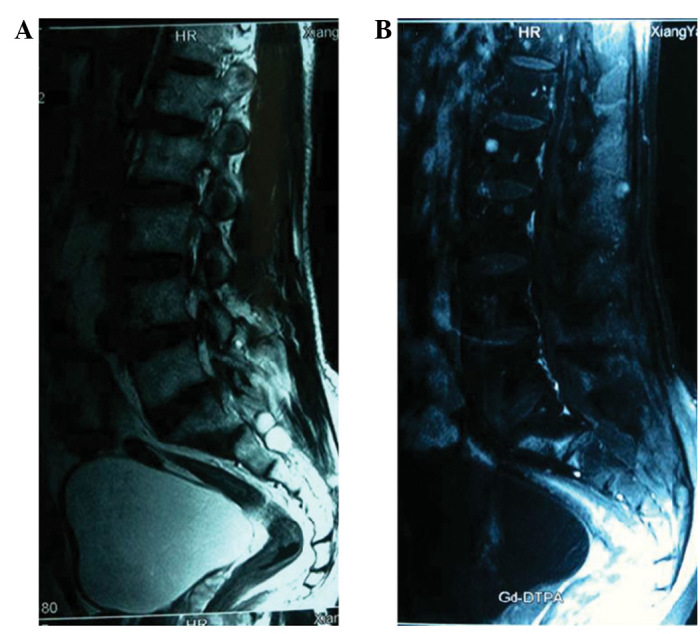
(A) MRI and (B) contrast-enhanced MRI revealing osteogenic bone metastases in the spine.
